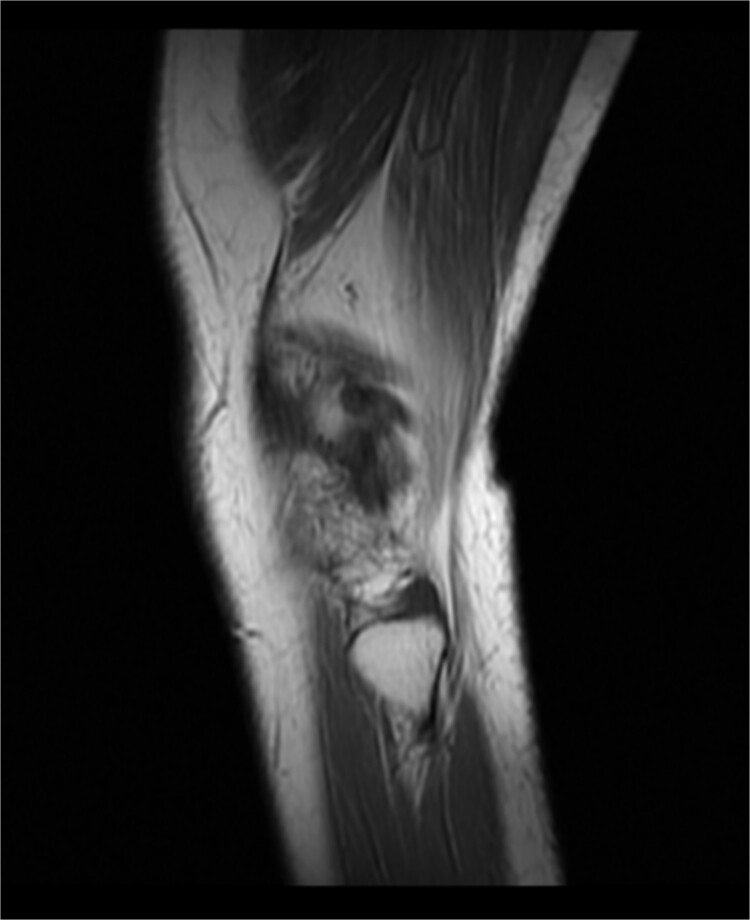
Pre-operation MRI image of the right knee joint of this patient.
